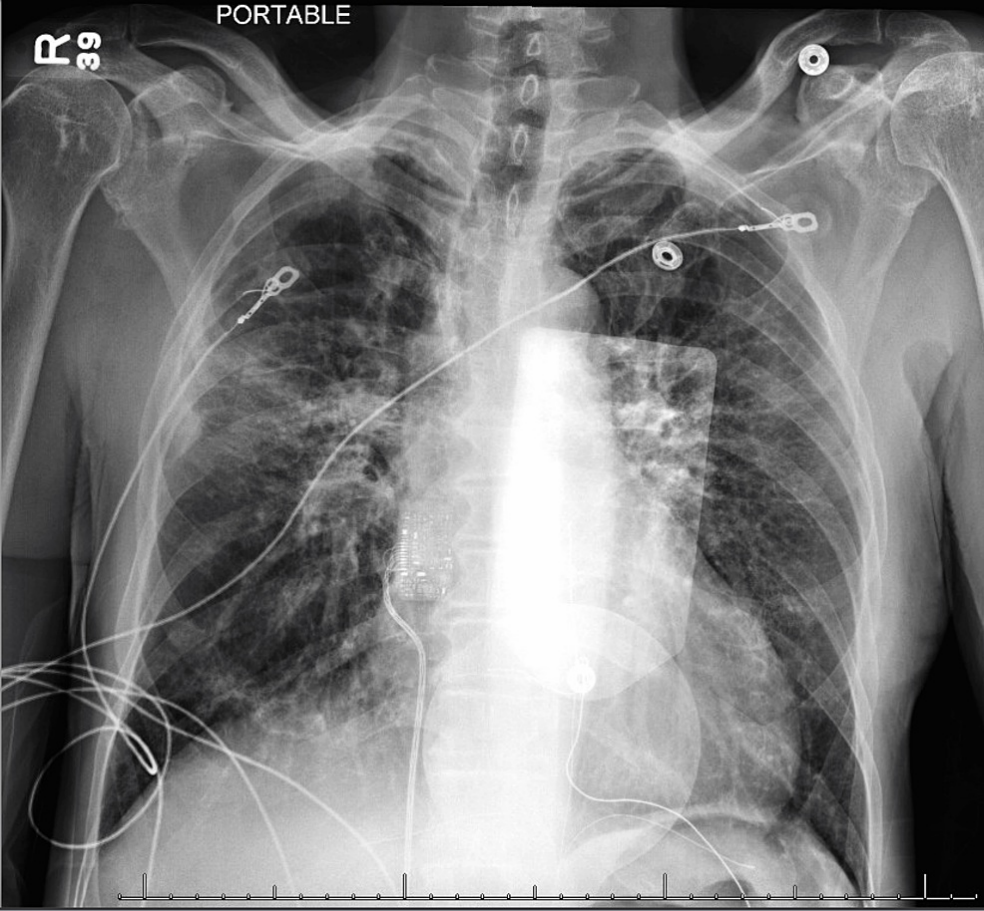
Chest X-ray with bilateral perihilar interstitial indistinctness and focal opacity in the right middle lung.
